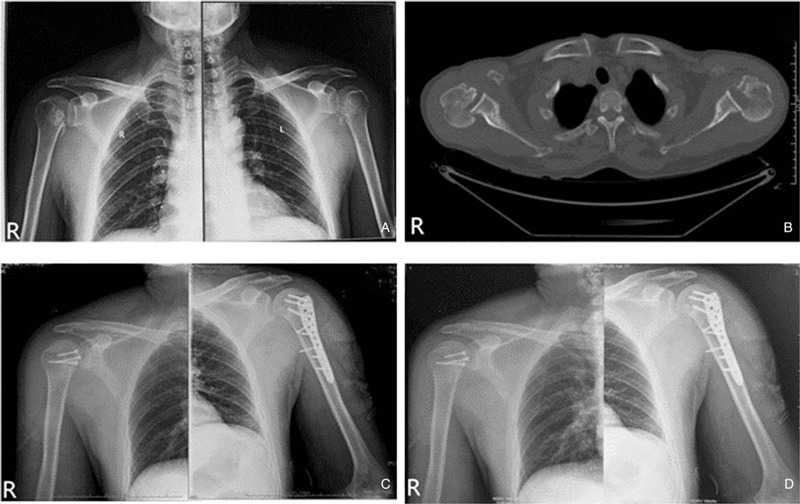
(a) Anteroposterior radiographs showing suspicious fractures of bilateral proximal humerus; (b) axial CT of both sides confirming the dislocation and showing a 3-part fracture on the left side and a 2-part fracture on the right side; (c) postoperative X-ray showing appropriate implants location; (d) X-ray findings after 16 months of follow-up showing good union and no avascular necrosis of the humeral head.
